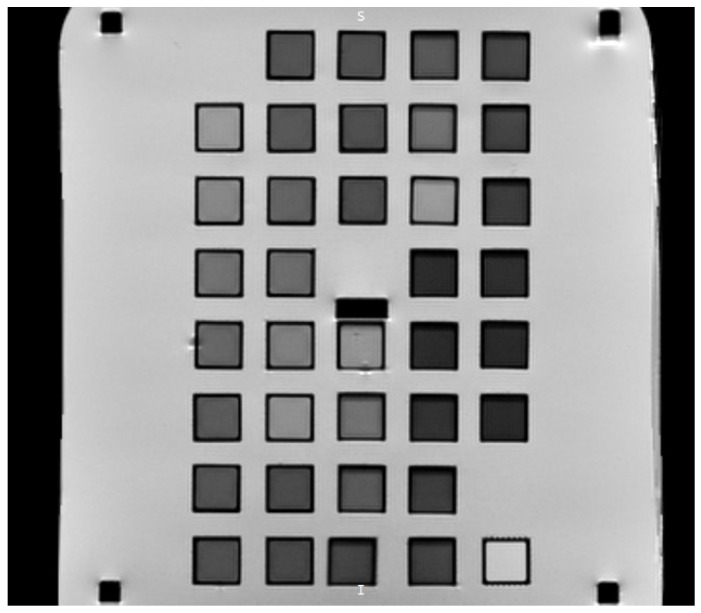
Cross-sectional MRI image of dosimetric gel samples.
